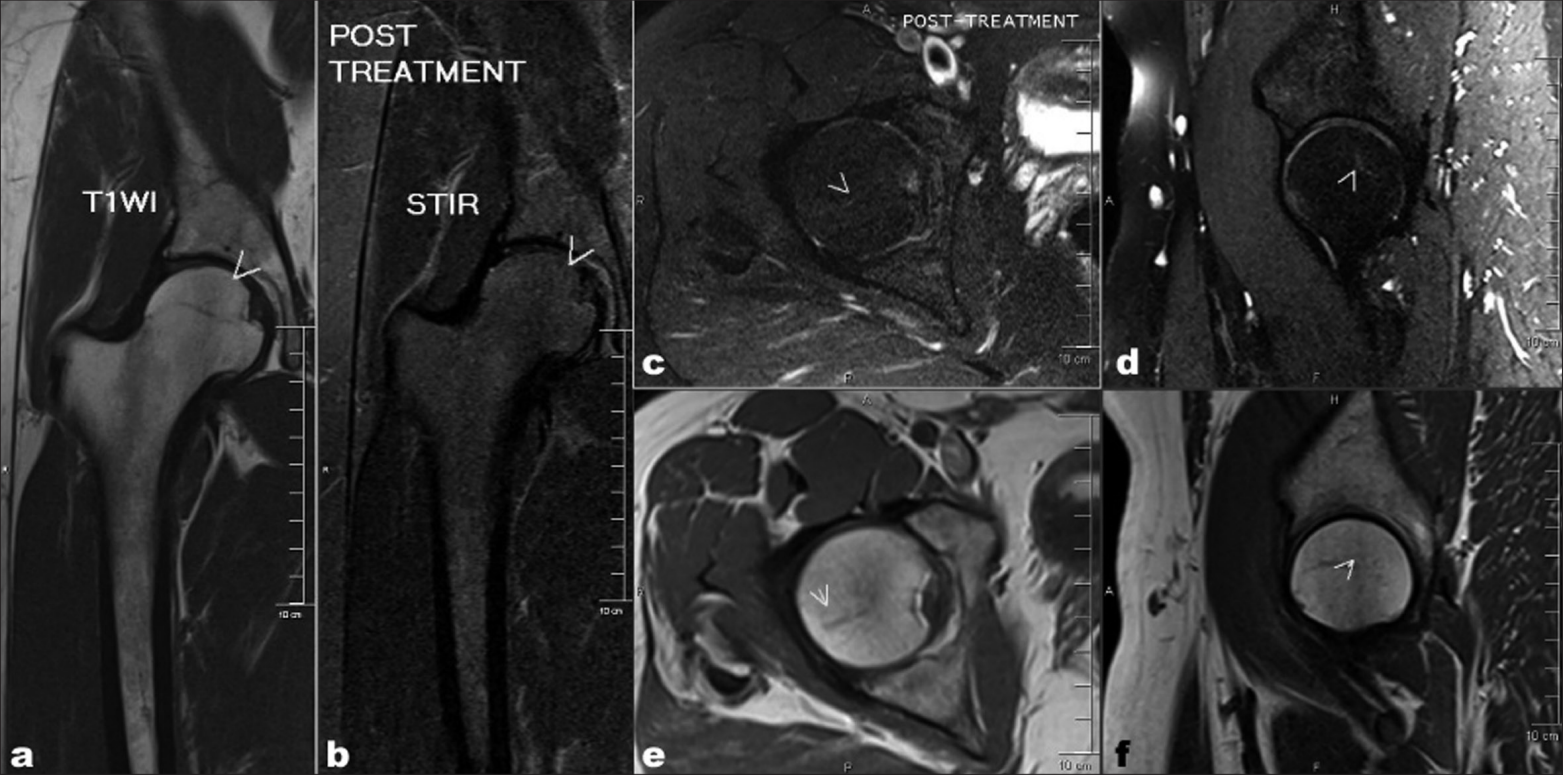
Coronal (a,b) and sagittal (c,d,e,f) magnetic resonance imaging (T-1 and STIR image) performed 8 weeks later showing resolution of bone edema and absence of fracture line (marked with arrowhead)
